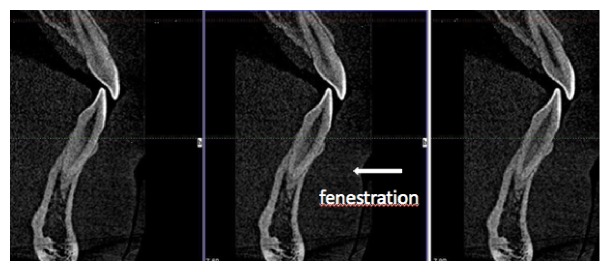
Cone-beam computed tomography (CBCT) images showing fenestrations in the area of the incisors.
